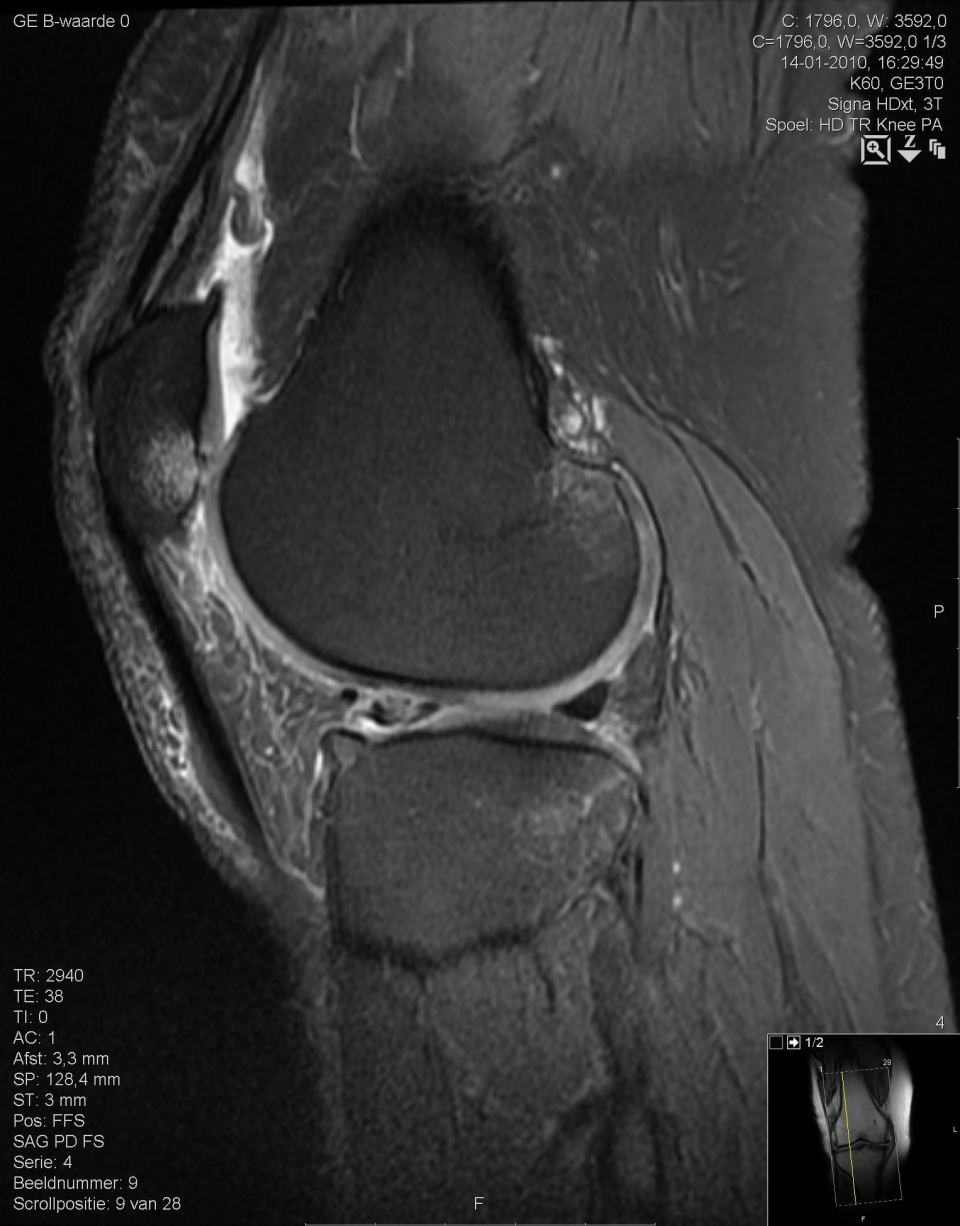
Infrapatellar synovitis. Fat-suppressed sagittal proton density-weighted turbo spin-echo 3.0 Tesla magnetic resonance imaging scan.
